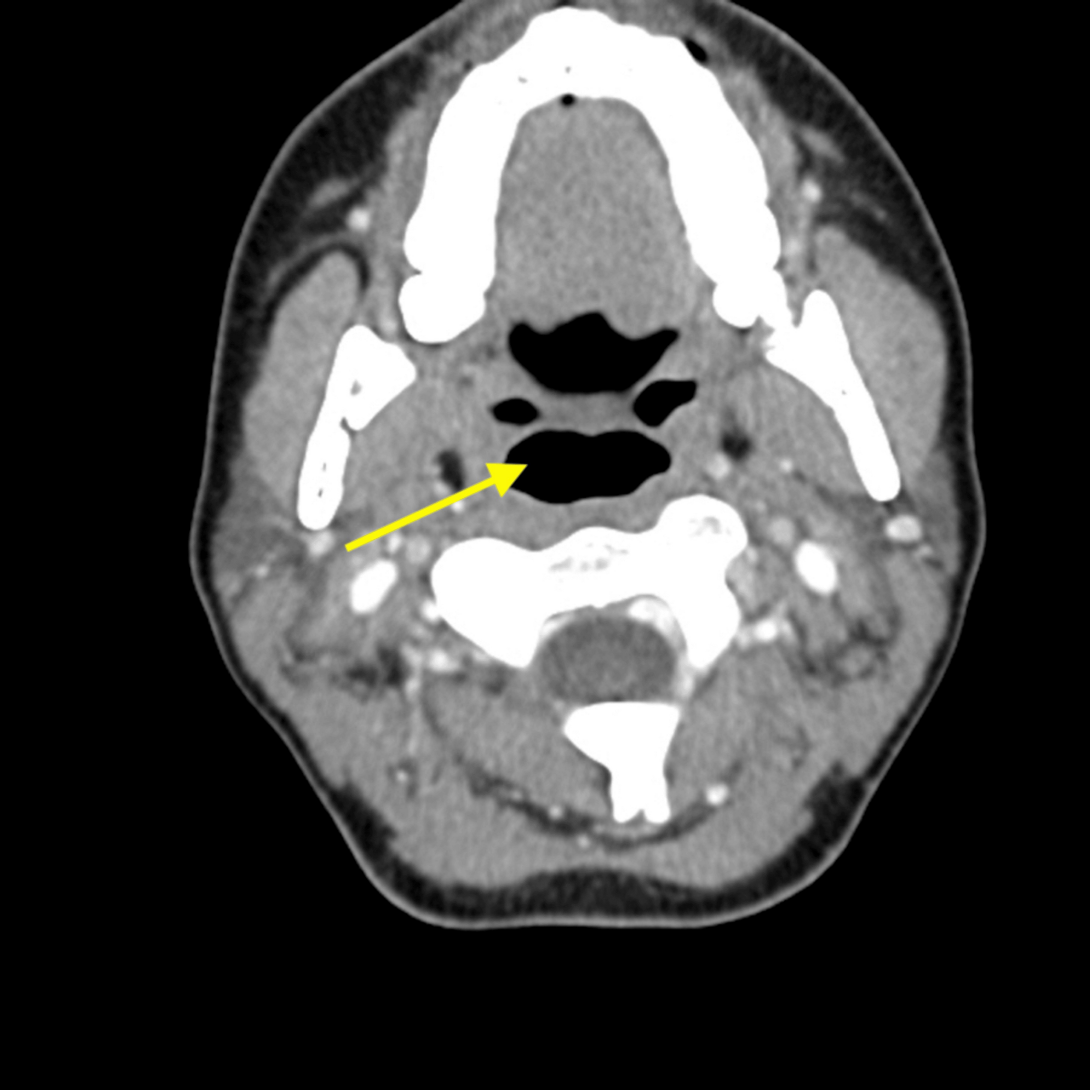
CT scan of neck, axial view Arrow demonstrating patent airway without evidence of obstruction.
